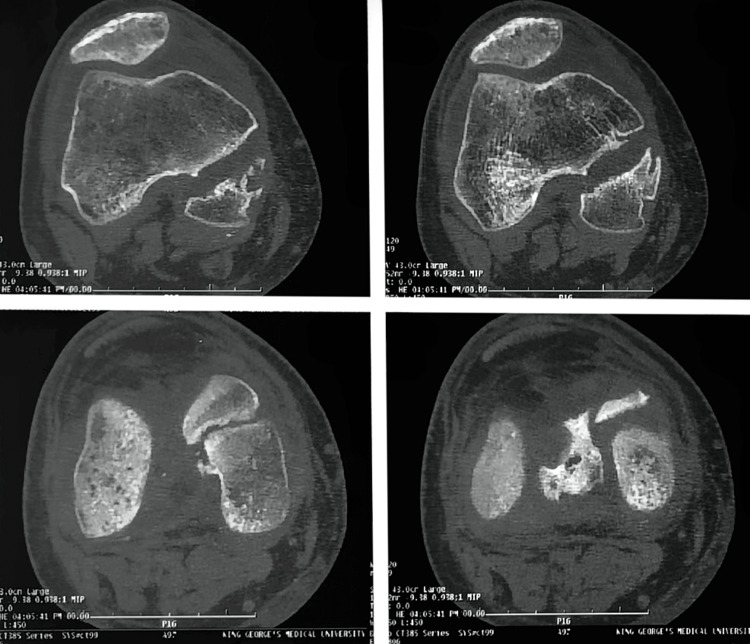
CT showing the medial condyle Hoffa fracture (R)
